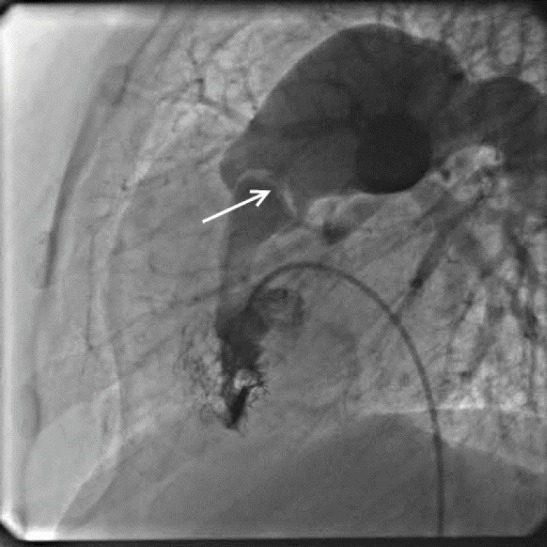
The patient was observed a few hours in the recovery room and was then transferred to the ward. After a few hours the patient gradually developed dyspnea and decreased oxygen saturation. Noninvasive oxygenation with facial mask was initiated. Her examination revealed blood pressure of 100/80 mm Hg, pulse rate of 128/min, respiratory rate of 28/min, cyanotic lips, diffuse bilateral fine rales over the lung fields and a harsh systolic murmur best heard in pulmonic area. Her blood gas analysis demonstrated mixed respiratory and metabolic acidosis and significant hypoxia and desaturation. A repeat echocardiography revealed trans-pulmonary valve gradient of 45 mm Hg associated with mild regurgitation without any other new findings. Portable chest X-ray revealed bilateral diffuse haziness (Figure 2). As she was unresponsive to non-invasive oxygenation with mask, she was intubated with endotracheal tube and transferred to the intensive care unit (ICU). In ICU she was ventilated with assist control mode with a rate of 12/min, tidal volume of 500 mL, partial oxygen fraction of 100% and PEEP of 5 mm Hg. Her 24-hour fluid intake was restricted to 1500 mL and she was given intravenous furosemide. Despite initial increase in arterial oxygen saturation, her condition remained critical with further deterioration in hemodynamics with decreased systolic blood pressure to 80 mm Hg and increased heart rate to 145/min. Her ICU course was further complicated by ventilator associated pneumothorax which was managed with chest tube insertion. Unfortunately despite all efforts, she died less than 3 days after being transferred to ICU due to shock state and severe oxygen desaturation.
